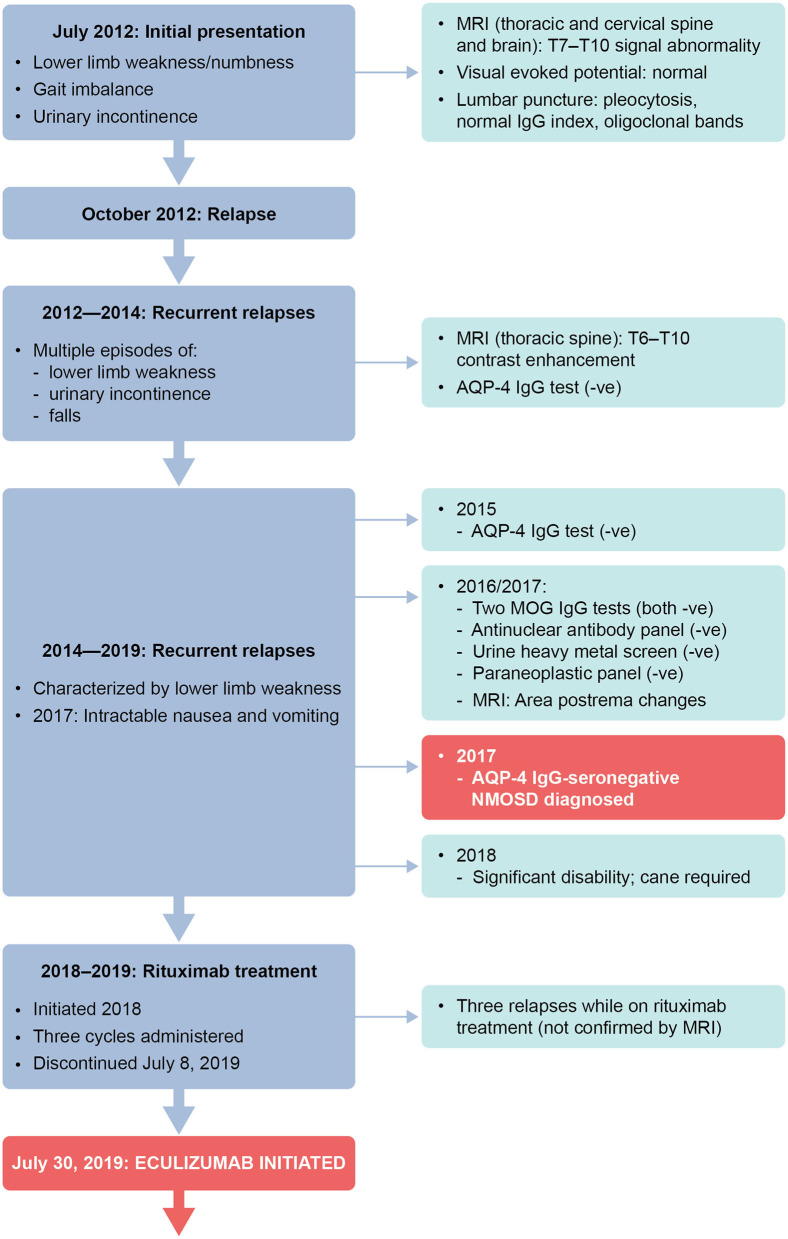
Timeline of clinical presentation and investigations. –ve, negative; AQP-4, aquaporin-4; IgG, immunoglobulin G; MOG, myelin oligodendrocyte glycoprotein; MRI, magnetic resonance imaging.
